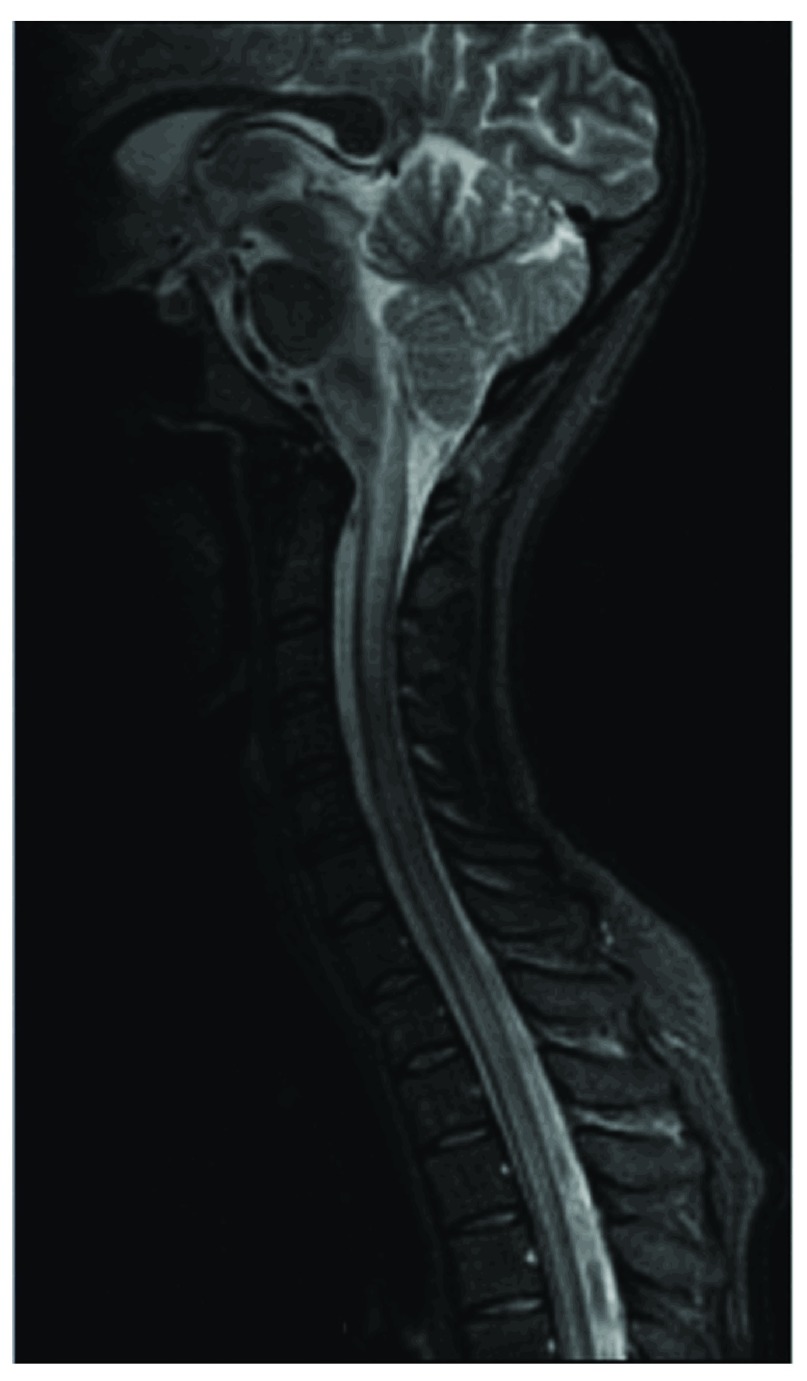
Sagittal T2-weighted image shows an extensive hyperintensity involving the medulla oblongata and the cervico-toracic cord, compatible with a myelitis pattern. Figure origin: Department of Radiology, Hospital Clinic Barcelona.
